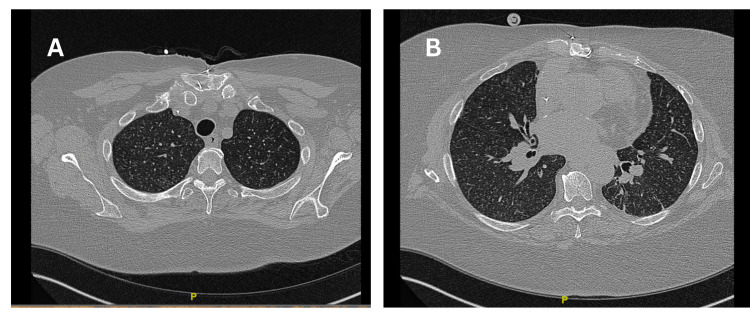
High-resolution computed tomography (HRCT) chest showing micronodular opacities in bilateral lungs.
